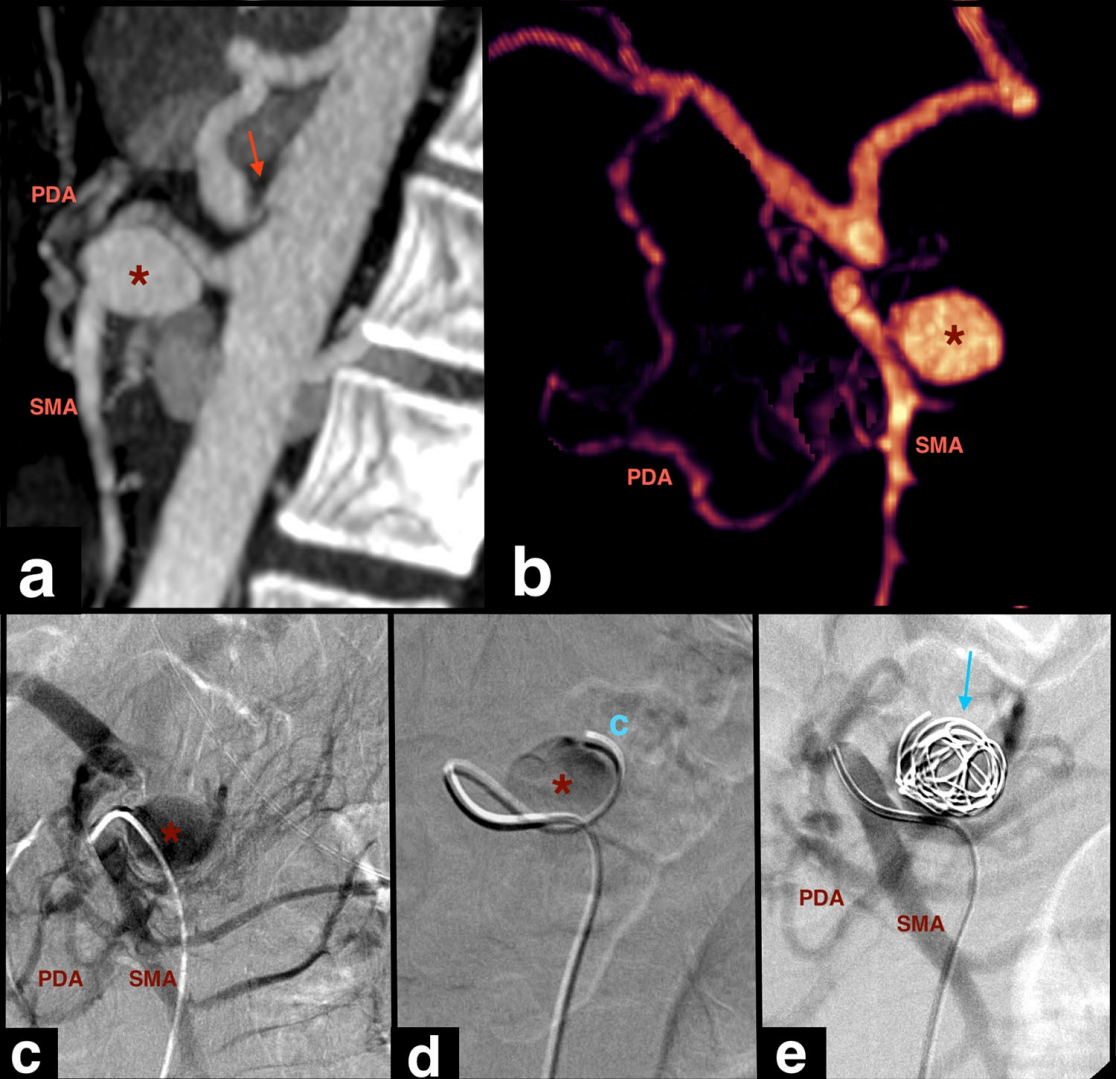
Aneurysm of the inferior pancreaticoduodenal artery in a patient with celiac artery stenosis. Sagittal MIP (a) and 3D MIP reconstruction (b) CTA images show the celiac artery compression (arrow in A), and the aneurysm (*) measuring 2 cm. Selective superior mesenteric artery (SMA) angiogram (c) shows the aneurysm (*). After selective catheterization with a microcatheter (c in image d), the aneurysm was embolized with coils (arrow in e), with prophylactic intent. CTA, computed tomography angiography; MIP, maximum intensity projection; PDA, pancreaticoduodenal artery
